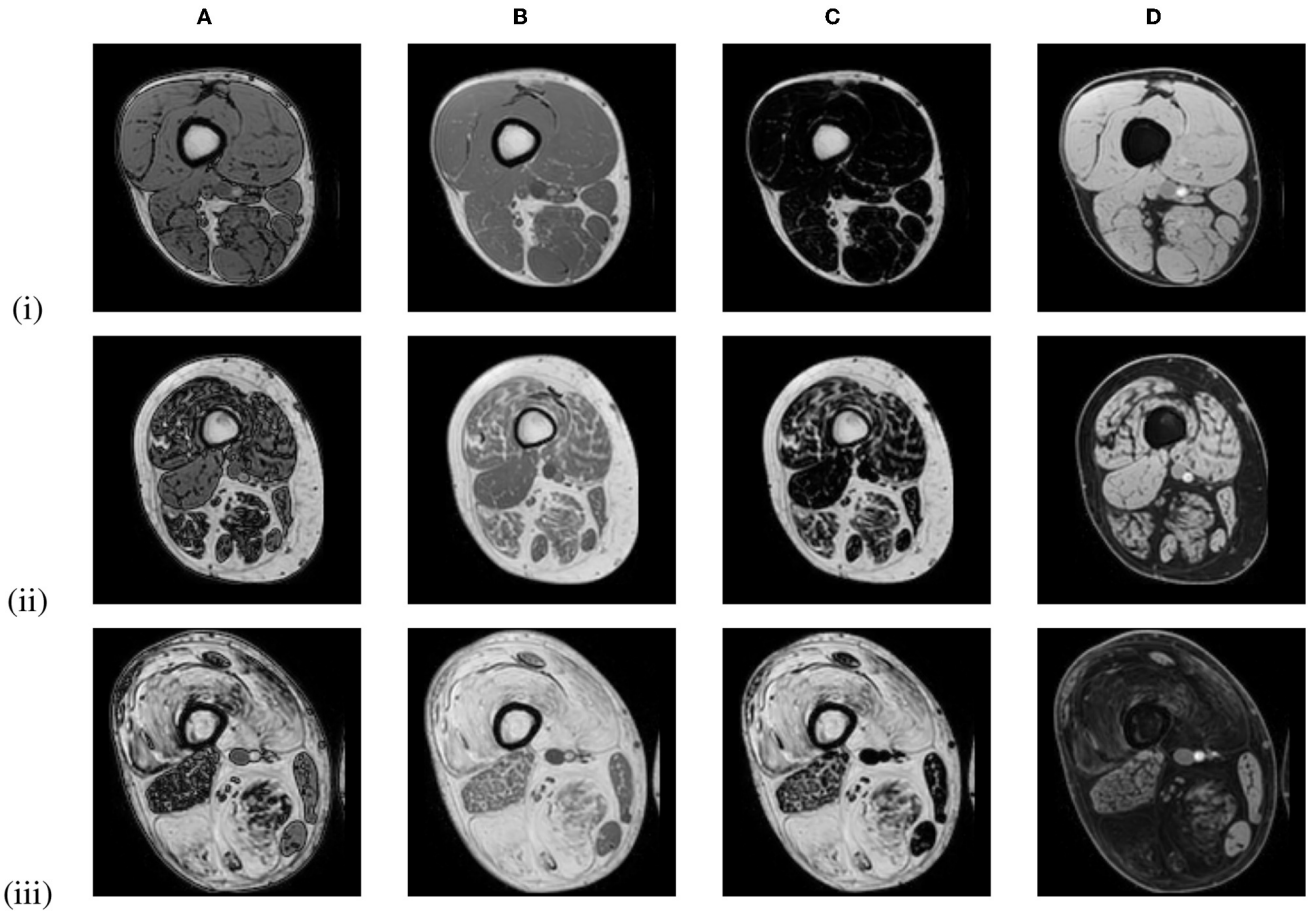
Out-of-phase (A), in-phase (B), fat-only (C), and water-only (D) Dixon MRI images of the proximal leg of 3 LGMD patients in an early-stage (i), an intermediate-stage (ii) and an end-stage (iii) of the disease, showing increased replacement of muscle tissue by fat.
